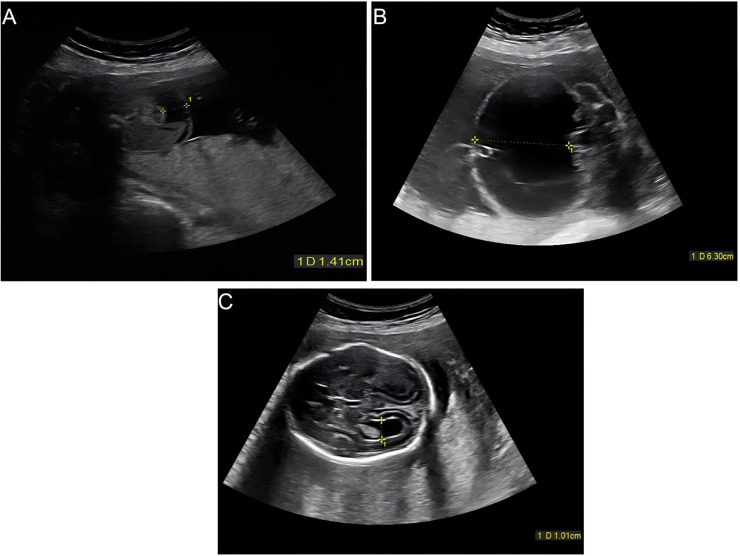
Serial prenatal ultrasound findings of congenital cytomegalovirus infection at 18 and 23 weeks of gestation. (A) Transabdominal ultrasound at 18 weeks showing echogenic bowel and mild fetal ascites (maximum depth, 1.4 cm). (B) Follow-up ultrasound at 23 weeks showing severe fetal ascites (maximum depth, 6.3 cm). (C) Ultrasound at 23 weeks showing ventriculomegaly, with the right lateral ventricle measuring 1.0 cm.
